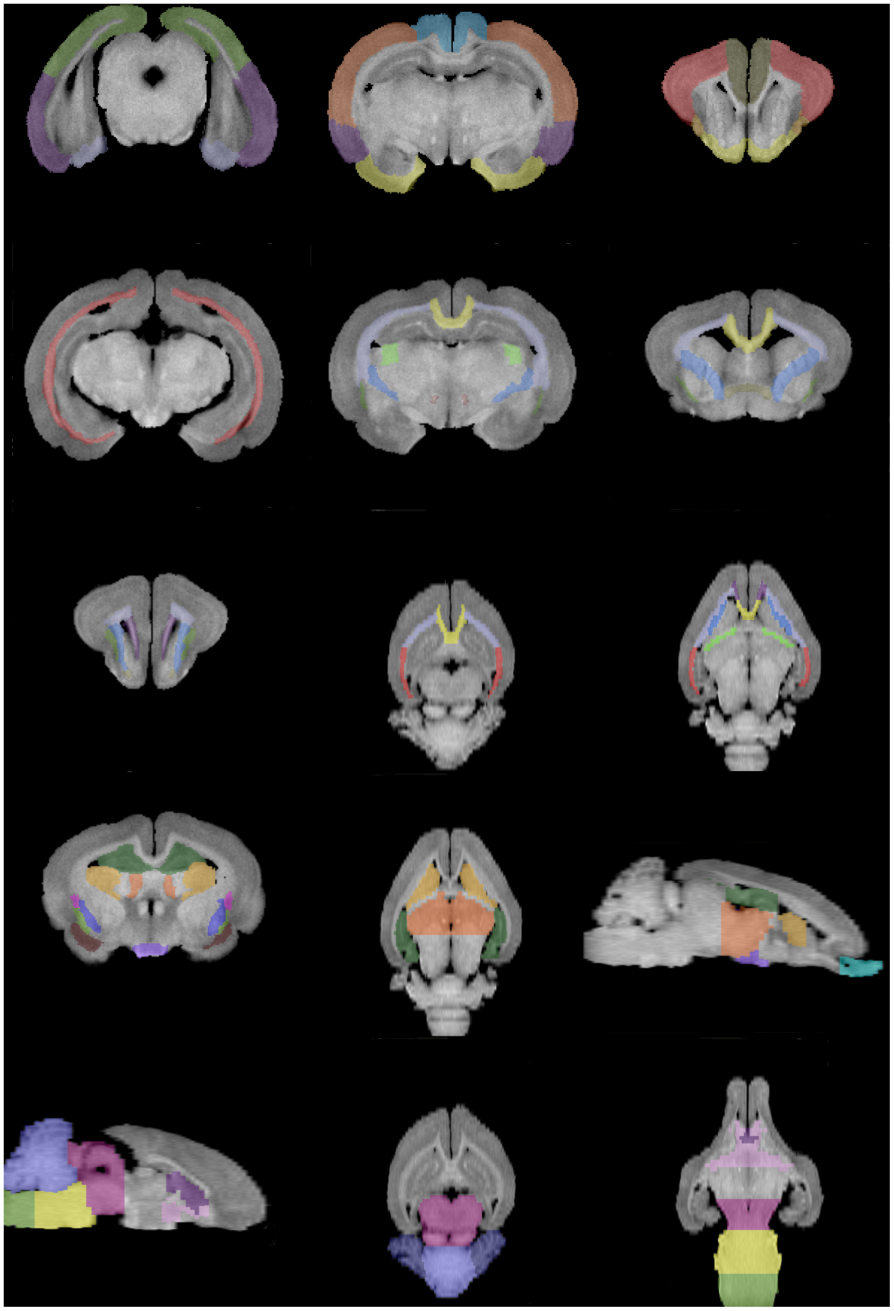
Brain regions overlapped over representative slices of the T1-weighted image. First row: cortical regions; second and third rows: white matter regions; fourth row: deep gray matter and fifth row: other regions.
